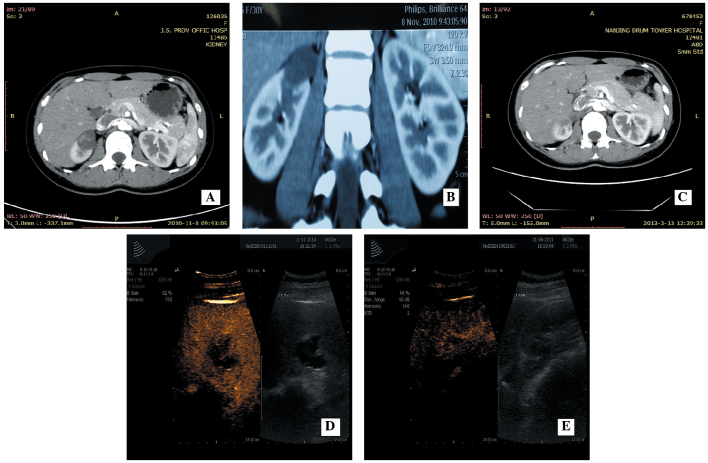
(A) Pre-operative computed tomography (CT) scan showing a 3.0×3.2 cm mass with cystic and solid components at the superior pole of the right kidney. (B) Pre-operative axial CT image. (C) Post-operative CT scan showing no evidence of recurrence. (D) Pre-operative contrast-enhanced ultrasonography showing focal enhancement in the cystic zone. (E) Post-operative contrast-enhanced ultrasonography showing no evidence of recurrence.
